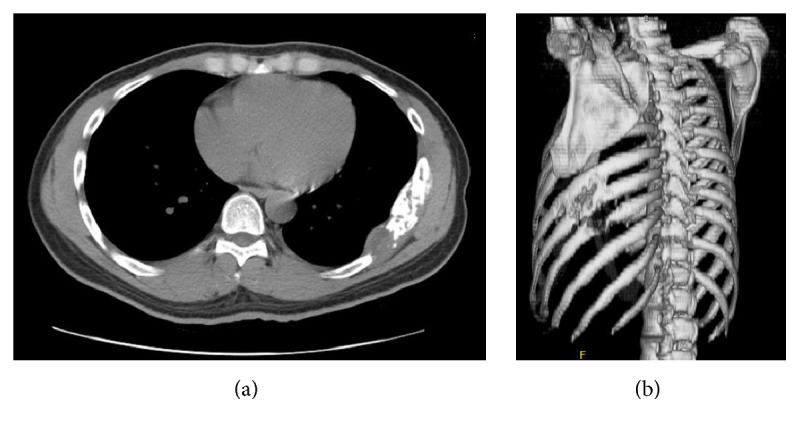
(a) Axial CT chest without contrast and soft tissue views demonstrating the destructive lesion involving the 7-8th ribs. (b) Three-dimensional CT reconstruction of the thoracic cage showing bony destruction of the 7th, 8th, and 9th ribs.
